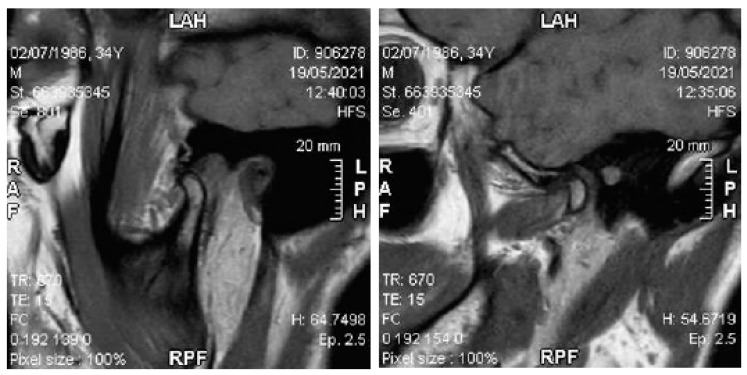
MRI images with a closed and open mouth.
